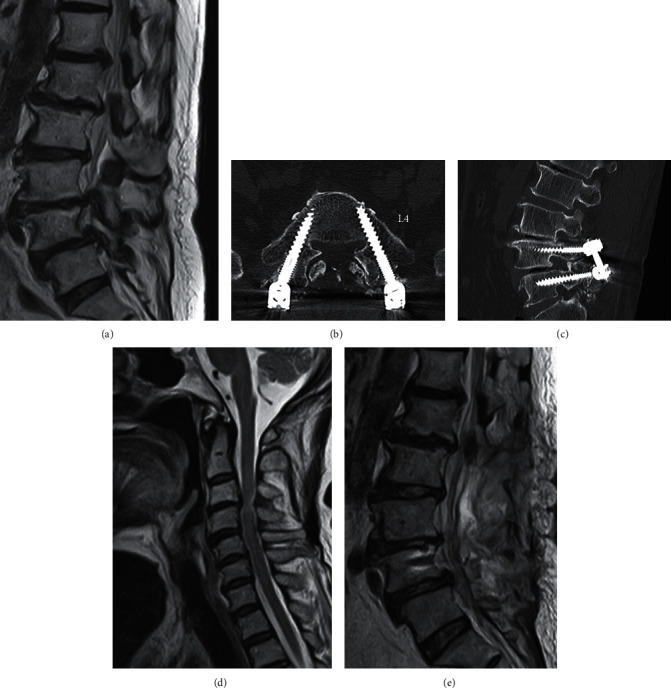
Imaging findings. (a) Lumbar magnetic resonance imaging (MRI) demonstrating severe lumbar spine stenosis at L1–5 and spondylolisthesis at L4/5. (b), (c) No percutaneous pedicle screw deviation or compression observed in the spinal canal. (d) Cervical MRI revealing spinal canal stenosis extending to C4–6 and disc herniation at the C3/4 level. (e) Lumbar MRI showing bilateral foraminal narrowing at the L4/5 level after laminectomy for L4 anterior spondylolysis, but no apparent implant deviation.
